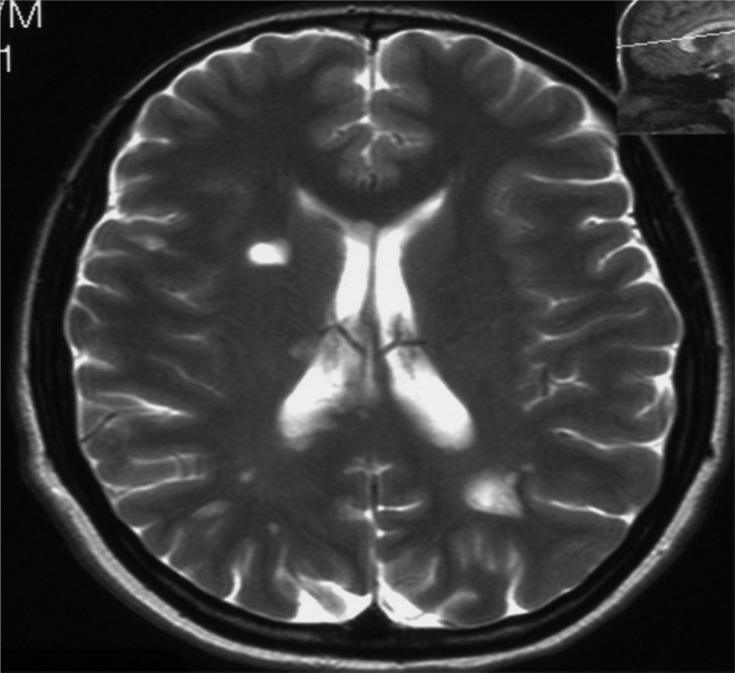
Brain magnetic resonance imaging, T_2_-weighted image showing hyper-signal demyelinating plaques in the periventricular and deep white matter areas
